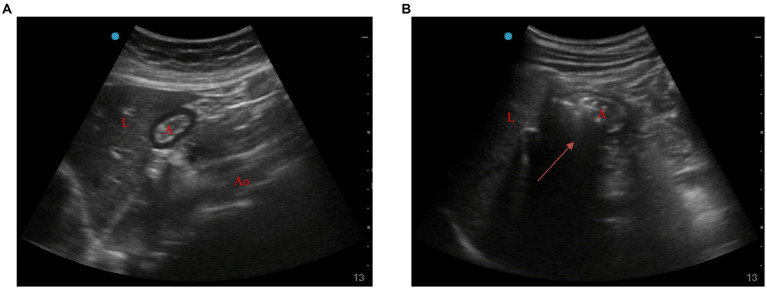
Ultrasonic images of gastric antrum before and after ventilation. (A) Before ventilation, (B) after ventilation (arrow). A, antrum; L, liver; Ao, aorta.
